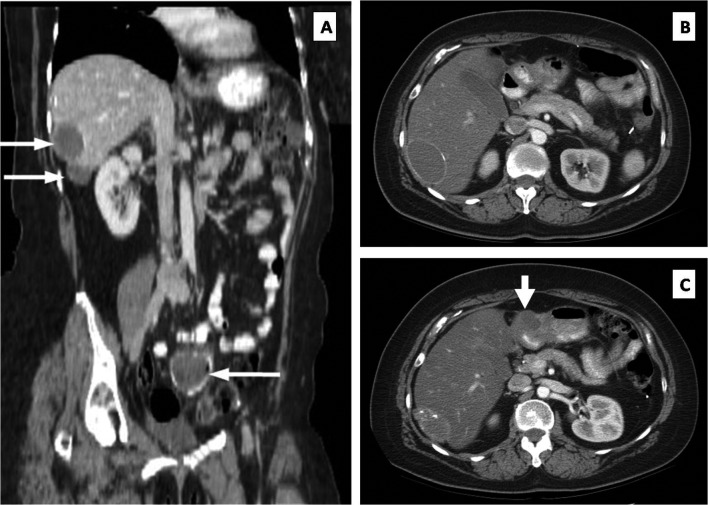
(A) Stable liver metastases (upper white arrows) and pelvic metastasis (lower arrow). (B) Axial CT showing no obvious peritoneal mass at the pylorus. (C) Repeat axial CT performed one year later documenting a new mass (thick white arrow) at the level of the pylorus.
